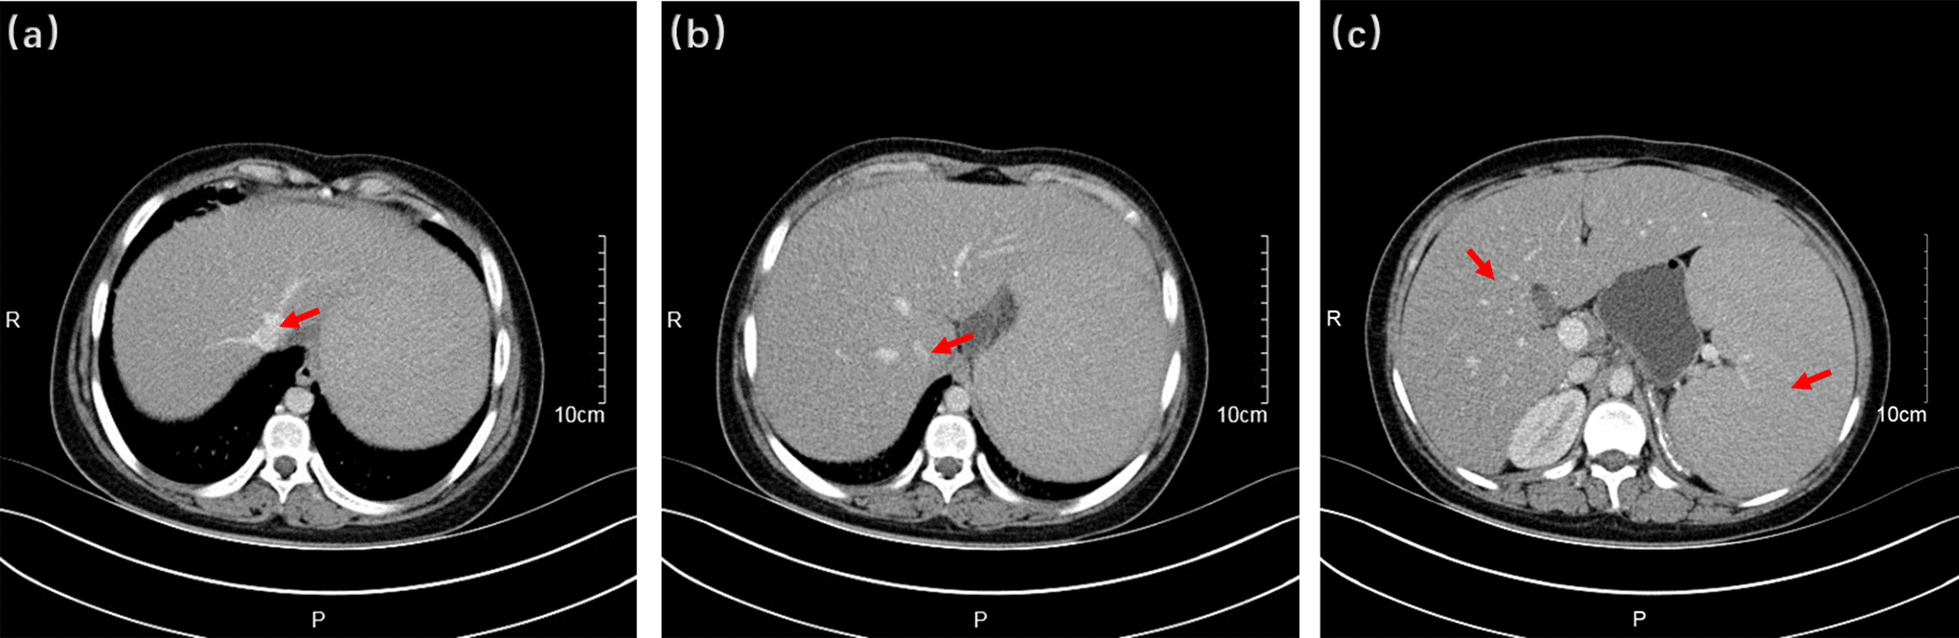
Enhanced CT of the upper abdomen revealed the place where the hepatic vein merged into the inferior vena cava was thin (a, b) and hepatosplenomegaly (c).
